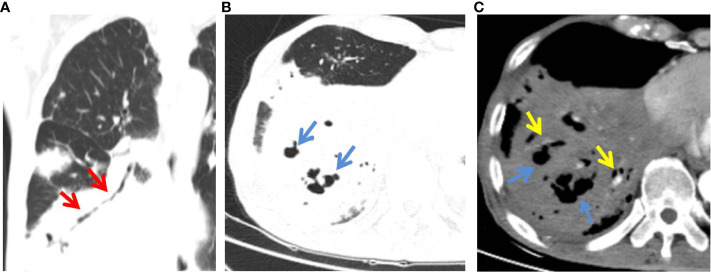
C-MALT lymphoma in a 58-year-old woman with right back pain for 2 weeks. (A) Lung-window HRCT multi-planar reconstruction showed a consolidation with air bronchogram (red arrow) in the right lower lobe. (B) There was cystic bronchiectasis (blue arrow) within the lesion. (C) Mediastinum-window showed consolidation with cystic bronchiectasis (blue arrow) and angiogram sign (yellow arrow). HRCT, high-resolution computed tomography; C-MALT, consolidation pattern of pulmonary mucosa-associated lymphoid tissue.
